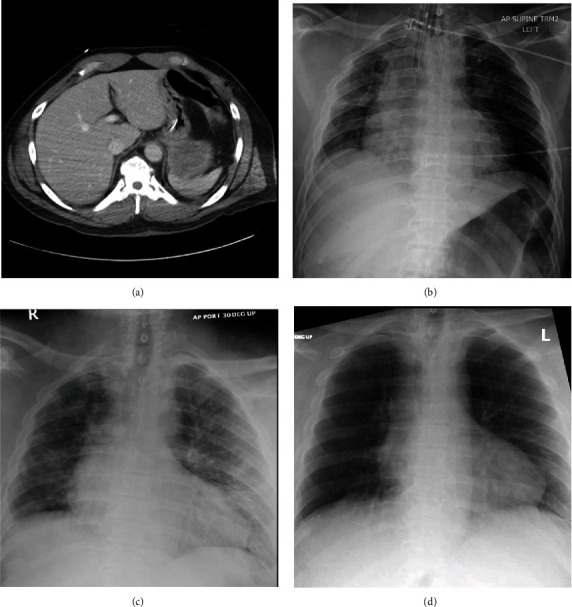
Patient 2 imaging including (a) admission abdominal CT with air pattern concerning for diaphragmatic injury, (b) admission chest X-ray, (c) chest X-ray after positive COVID test, and (d) chest X-ray from the time of discharge.
